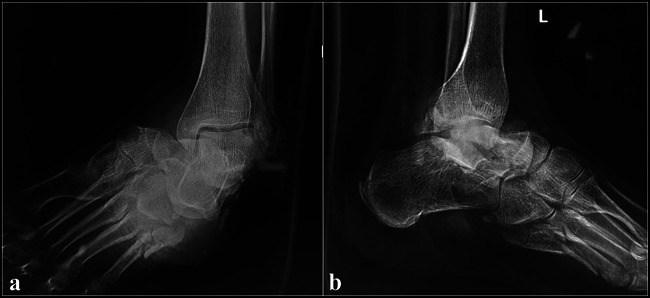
Radiographs of the medial subtalar dislocation case (presented in Figure 3). A, AP view. (B) Lateral view. Medial dislocation of the foot under the talus and absence of congruence of the talonavicular and subtalar joints are noted. The comminuted fifth metatarsal fracture is also noted.
